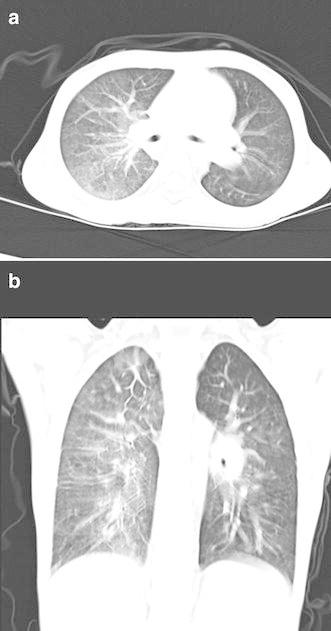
a The cross section of CT shows bilaterally increased pulmonary vasculature, especially in the right side. b The coronal plane shows bilaterally increased pulmonary vasculature, especially in the right side
